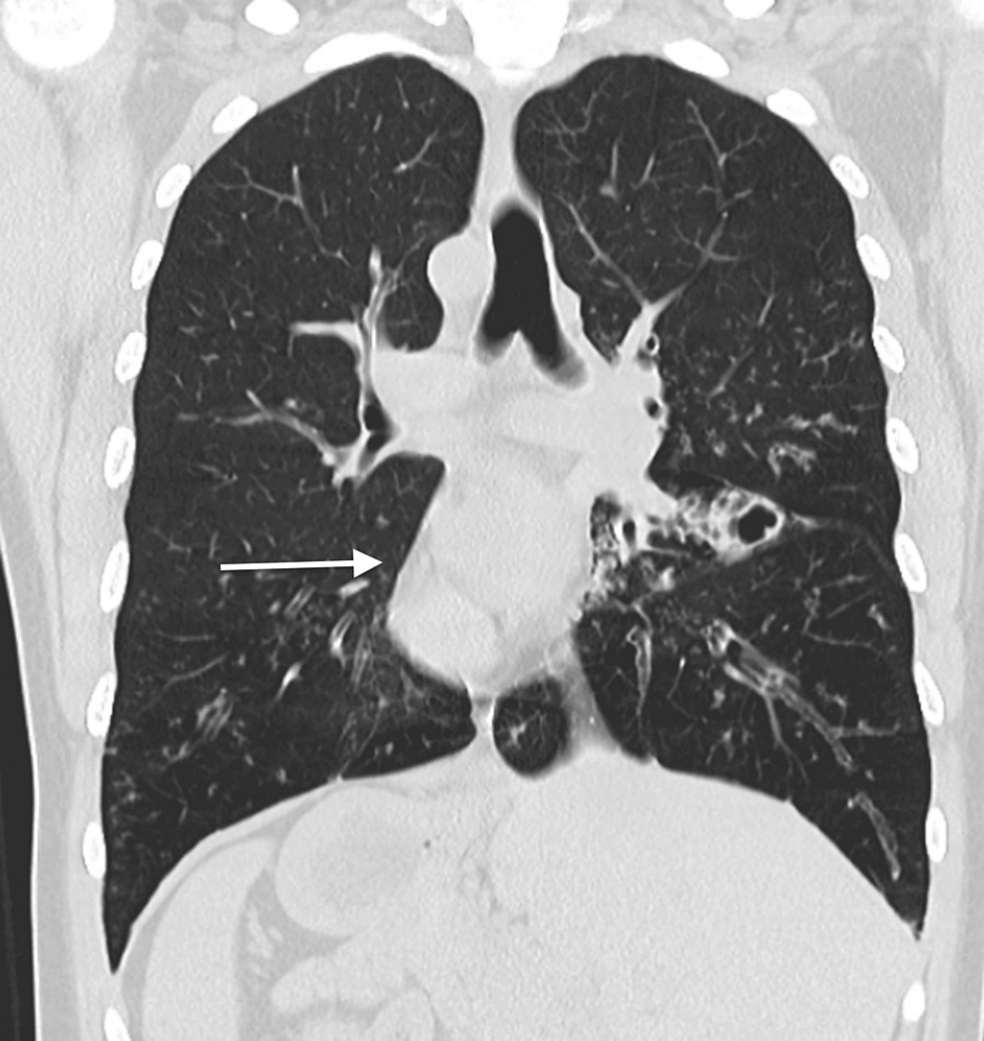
High-resolution computed tomography (HRCT) High-resolution computed tomography (HRCT) scan of thorax revealing bronchial wall thickening, cystic cavitary lesions in bilateral lungs and dextrocardia. Furthermore, the liver is seen on the right side and spleen on the left, suggesting situs inversus totalis.
